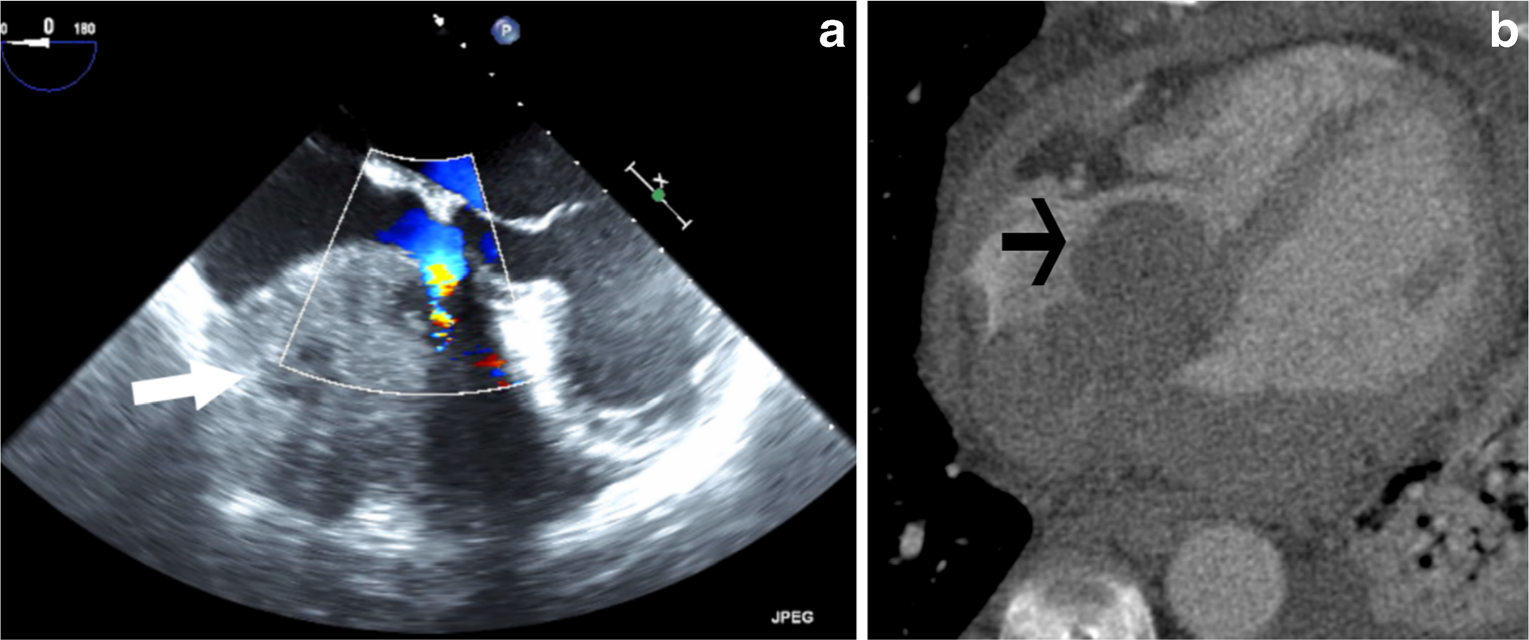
Angiosarcoma. (a) Echocardiogram shows a vascular tumour located in the right atrium (RA) (*arrow*), with displacement of the tricuspid leaflet and flow acceleration in the tricuspid valve. (b) Axial contrast-enhanced CT image shows a broad-based mass arising from the tricuspid valve (*arrow*), with involvement of the RA, interatrial septum and left atrium, and extension outside the heart into the pericardium
